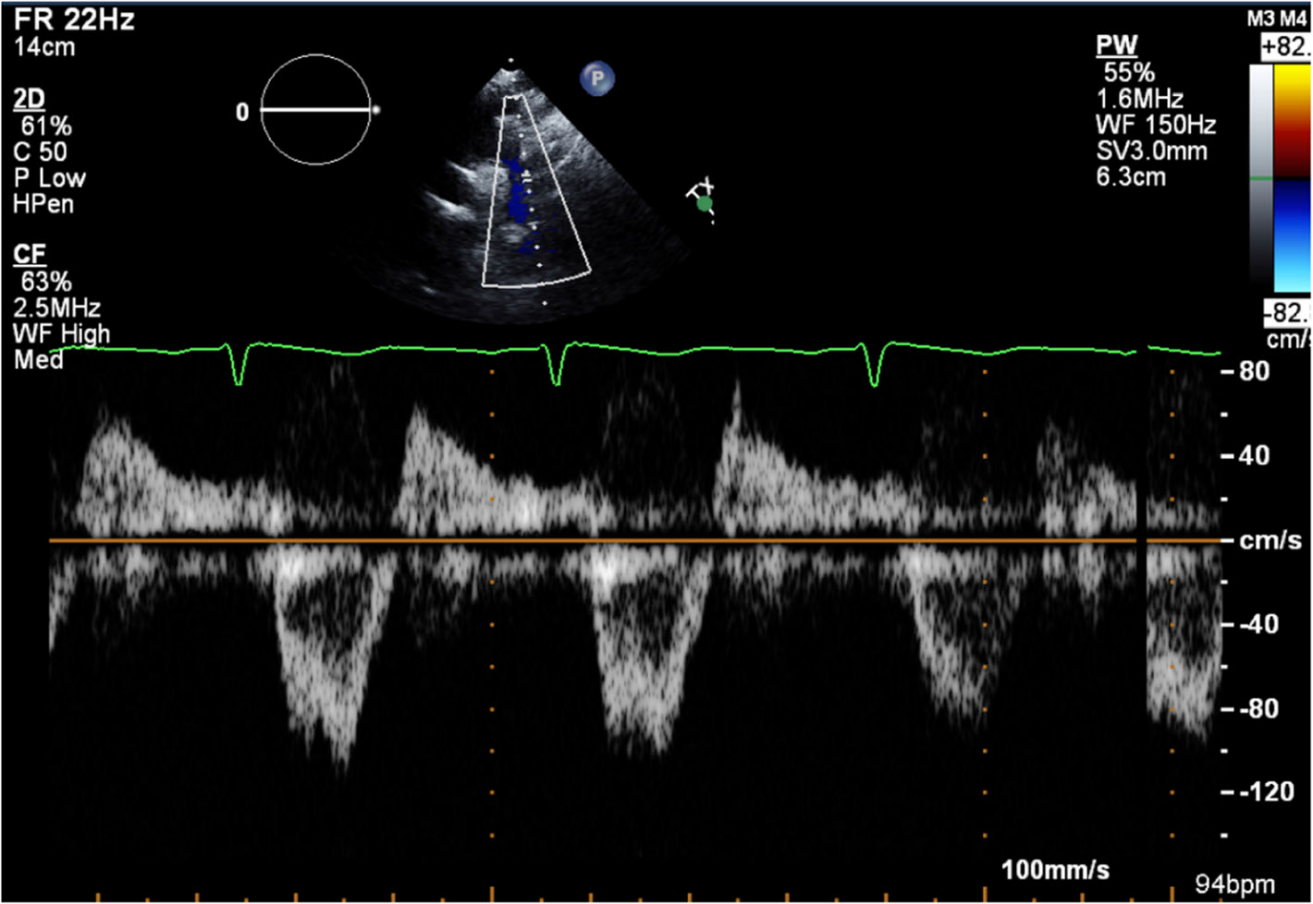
Transthoracic echocardiography suprasternal view with pulsed Doppler echocardiography demonstrating diastolic flow reversal in the descending thoracic aorta without evidence of aortic regurgitation
